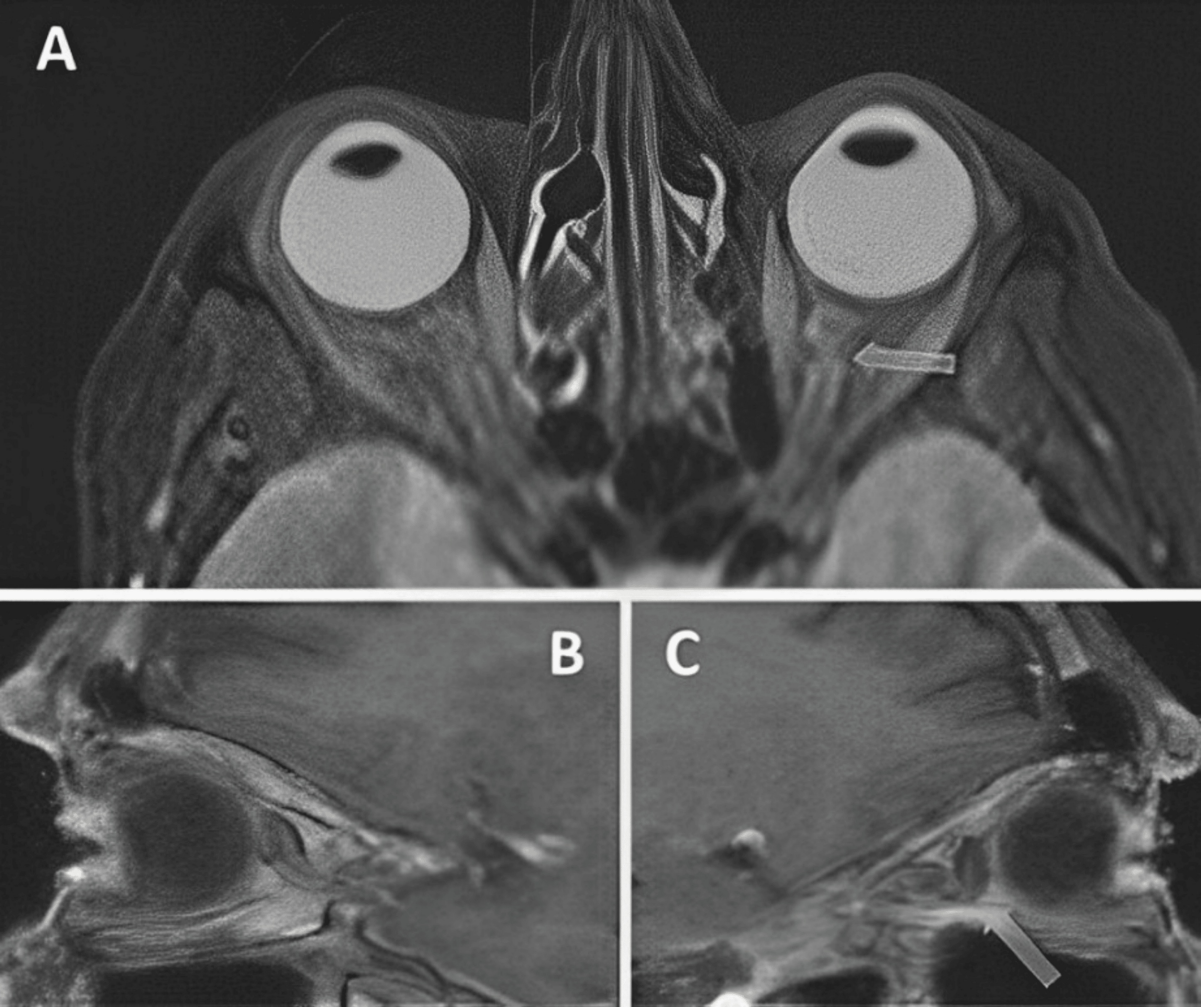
MRI showcasing the optic nerves. A: T2-oriented transverse MRI scan of the orbits, showing the kinking of the retrobulbar optic nerve in both eyes, more pronounced in the left eye (arrow). B, C: T1-oriented sagittal MRI scans of the right (B) and left (C) orbits, showing the kink in the retrobulbar course of the left optic nerve (arrow).
